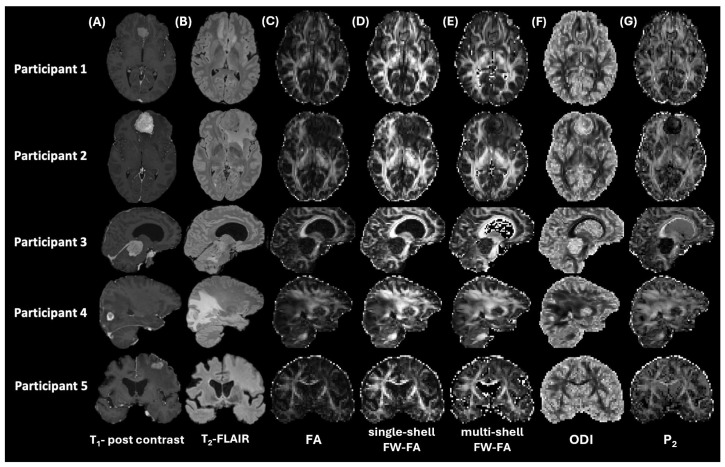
Representative (A) post-contrast T_1_-weighted images, (B) T2-weighted FLAIR images, (C) FA maps, (D) single-shell FW-FA maps, (E) multi-shell FW-FA maps, (F) ODI maps, and (G) P_2_ maps are shown. Post-contrast T_1_-weighted images best reflect tumor location, and T_2_-weighted FLAIR images best reflect tumor plus edema location. DTI’s FA (where WM is typically represented by a brighter signal intensity) fails to identify WM tracts through regions of edema (seen as hyperintense signal traced in T_2_-FLAIR images), whereas NODDI’s ODI (where WM is represented by a darker signal intensity) retains WM structure irrespective of edema presence. Similarly, the SM’s P_2_ map (where WM is typically represented by a brighter signal intensity) succeeds. Representative image planes were chosen on a per-patient basis to best reflect the lesion.
